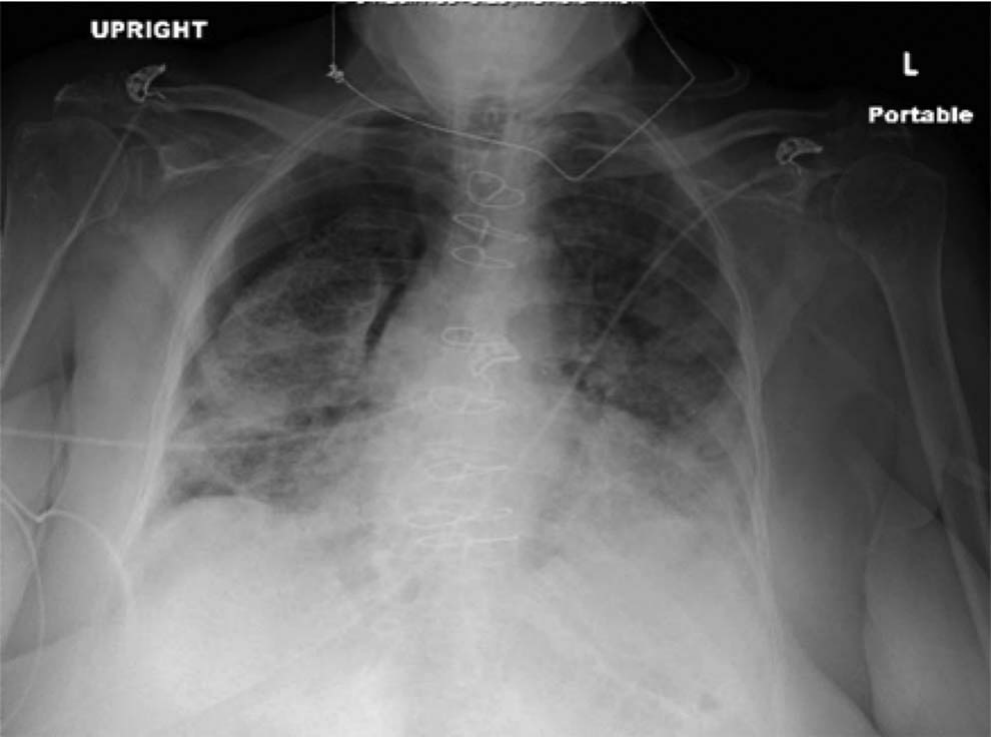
Chest radiograph revealed a right-sided 30% to 40% pneumothorax.
